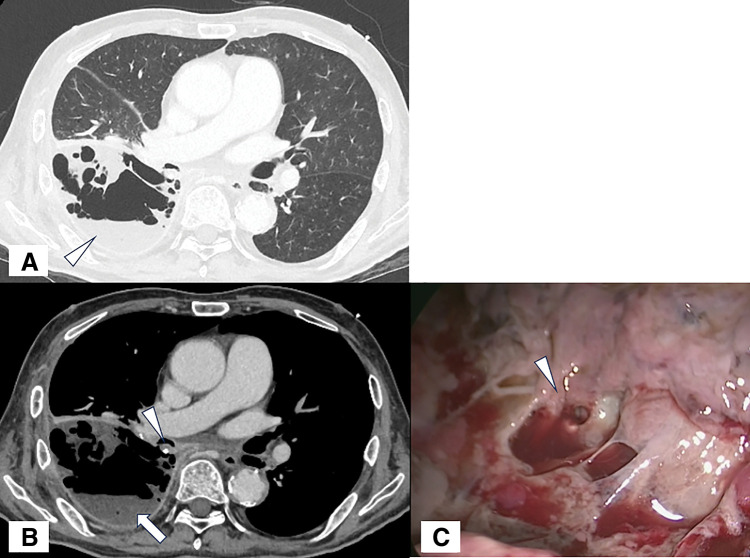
Findings of a bronchial stump fistula. (A) On lung window CT, lung collapse and pleural effusion (arrowhead) were observed. (B) On mediastinal window CT, pleural effusion and air accumulation in the right thoracic cavity (arrow) were observed, along with findings suggestive of communication between the bronchial and pleural cavities (arrowhead). (C) An approximately 1.5 cm fistula in the bronchial stump (arrowhead).
